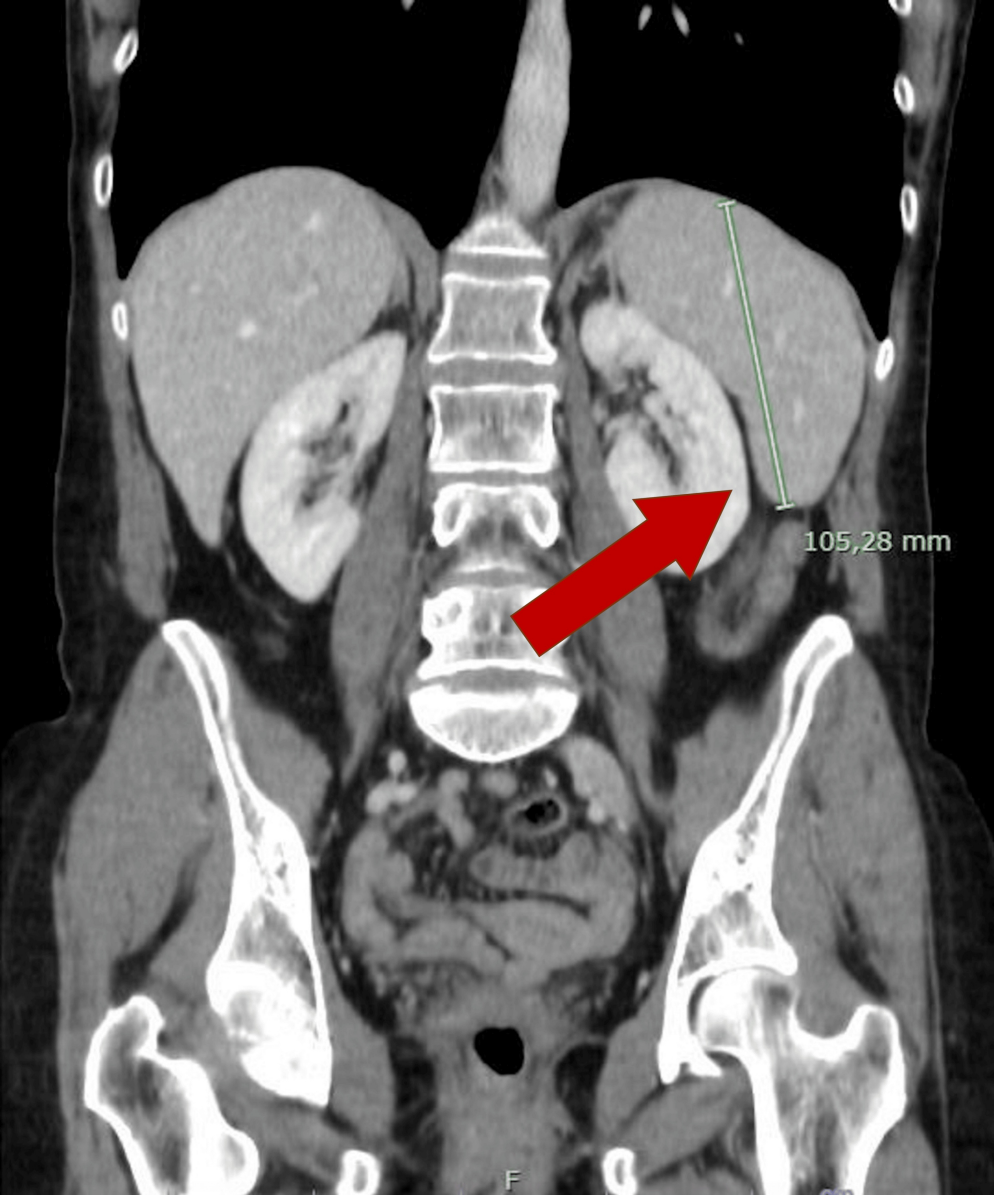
CT scan before T-DM1 treatment was started, showing normal spleen size (red arrow).
